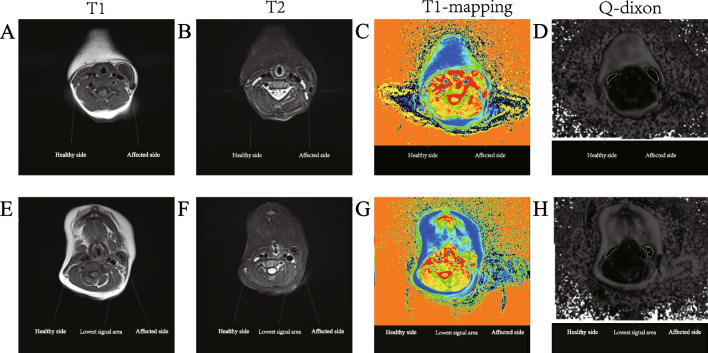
MRI imaging data and muscle texture data. The region of interest in the sternocleidomastoid of both sides was manually outlined, and T1WI, T2WI, T1-mapping, and Q-dixon sequences were obtained (a–d, respectively). The lowest signal area is seen in the affected SCM (e–h)
